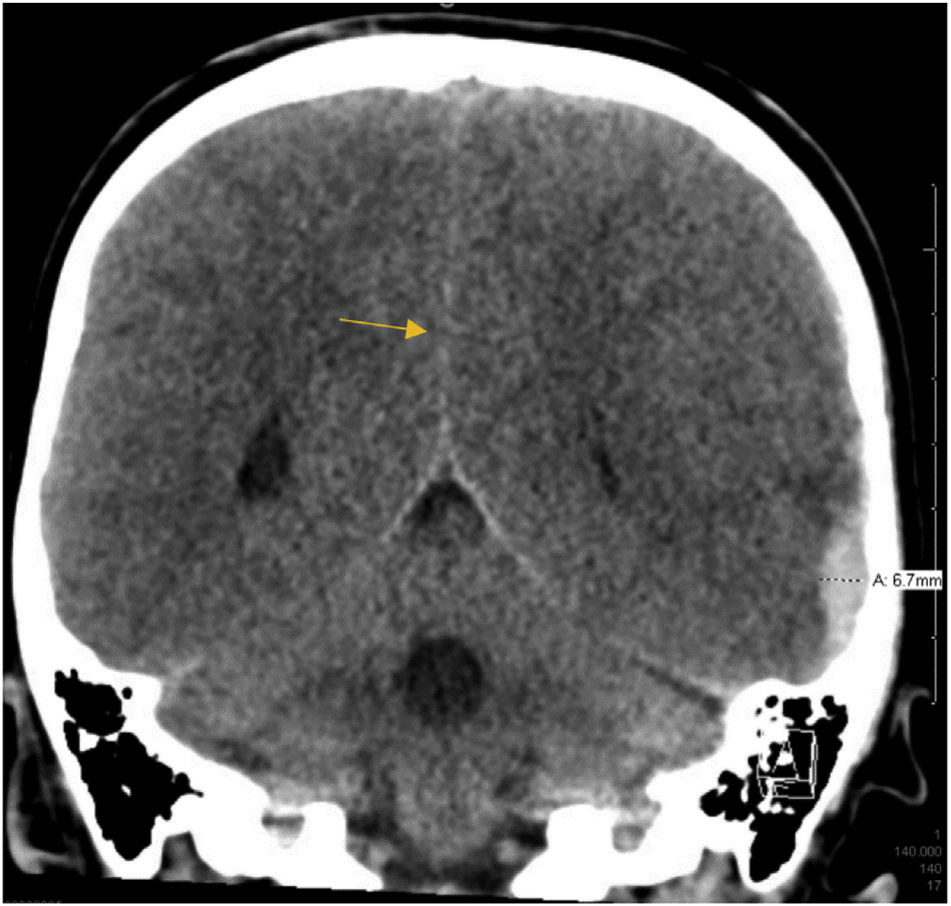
CT scan taken on seventh day of hospital admission indicates absence of midline shift.
